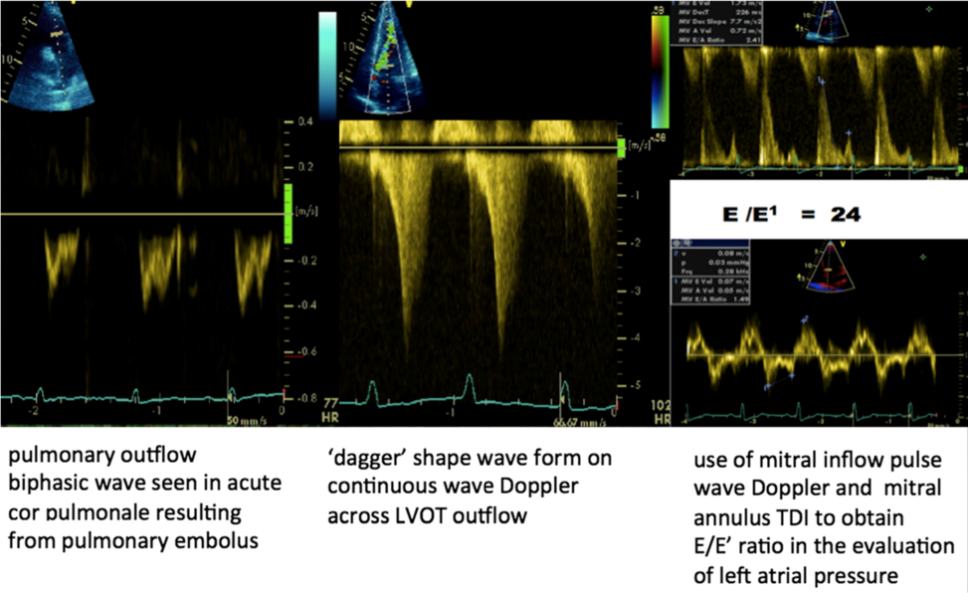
Examples of assessing the shocked patient using spectral Doppler
